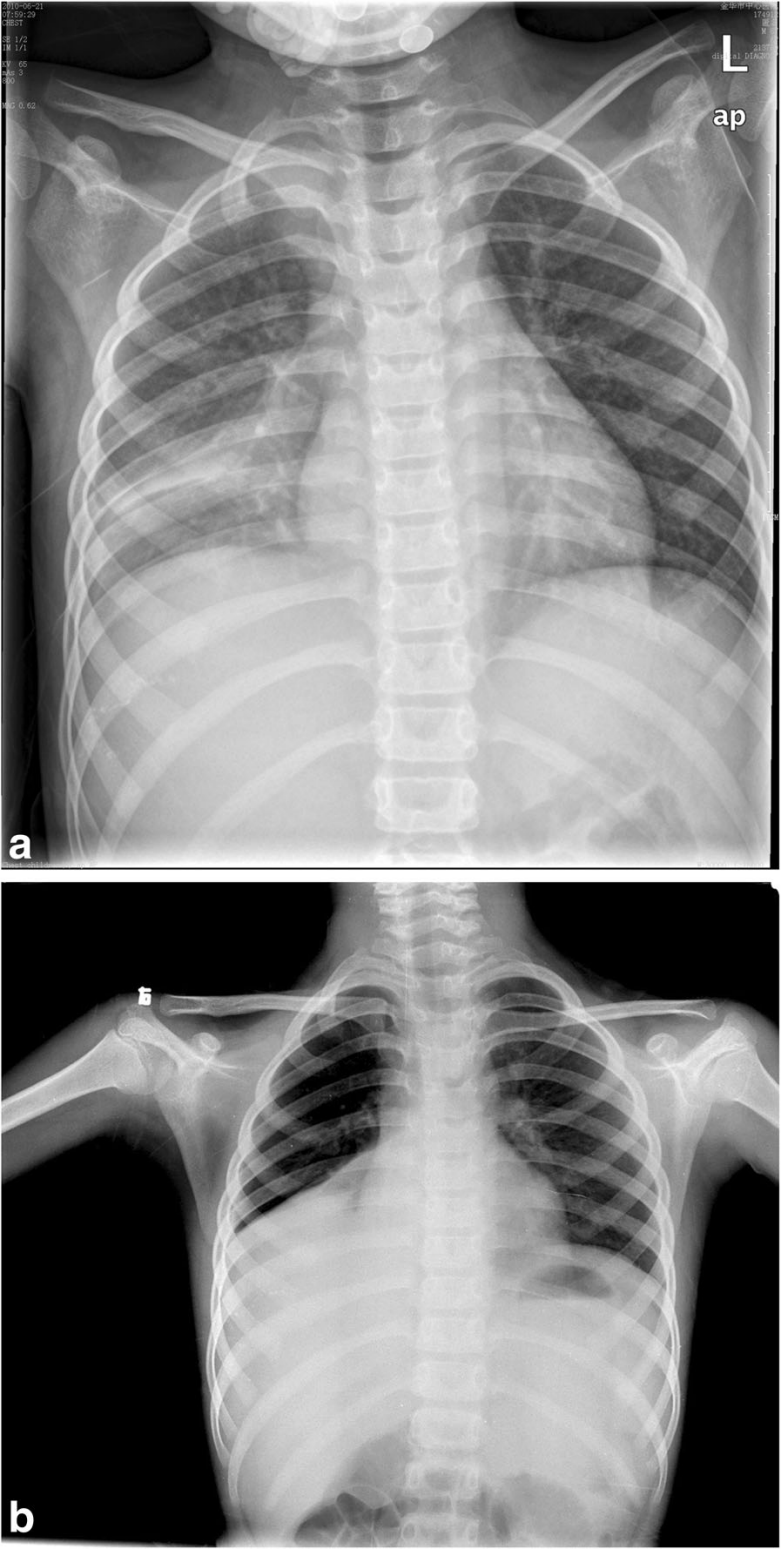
a Chest X-ray showed that two lung markings were increased, the high density lower right lung patchy shadows and a small right-sided pleural effusion at the initial presentation. b Chest X-ray showed that two lung textures were increased, the right lower lung had a high patchy density, and its edge was smooth. The right rib diaphragm angle was lost. The heart shadow had no obvious increase, and the left diaphragm was normal on the second day after transfer
